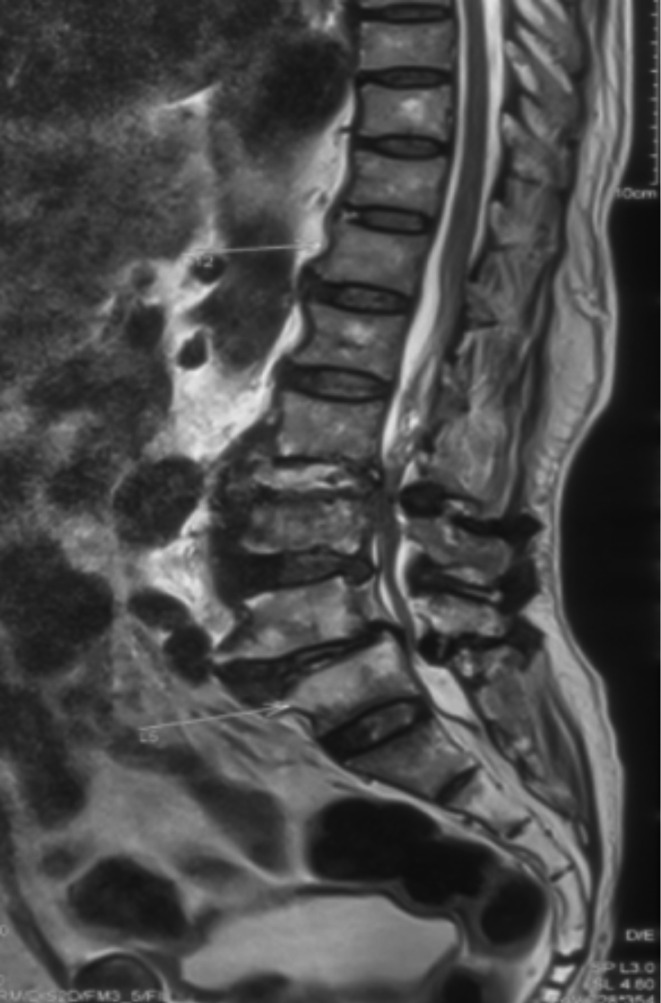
Magnetic resonance imaging T2 sagittal image showing features suggestive of spondylodiscitis at L2–3.
